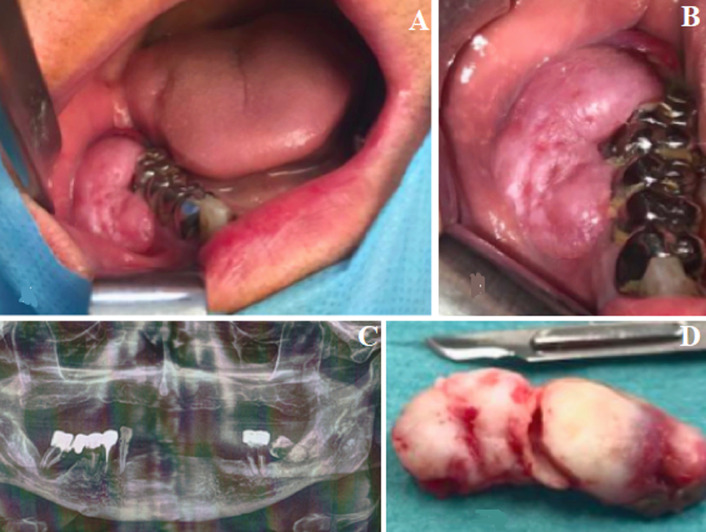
A,B) examen endobuccal initial; C) orthopantomogramme initial; D) pièce opératoire
